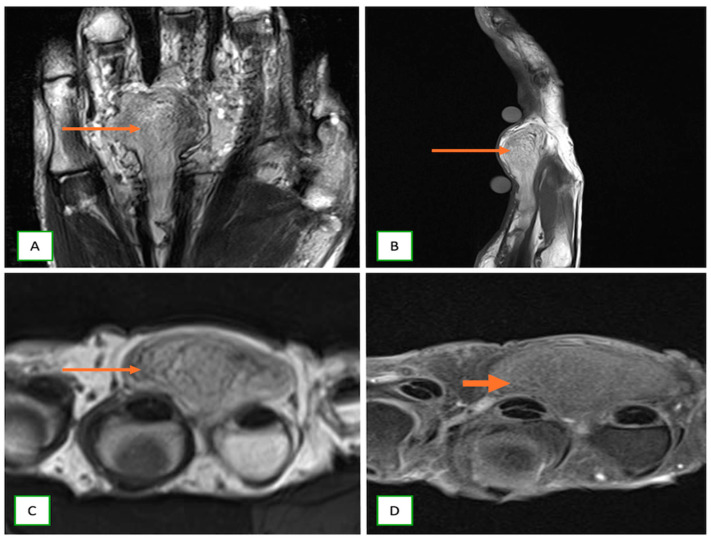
Lipofibromatous hamartoma of a digital nerve in a 53-year-old woman with a lump in their right hand. Coronal T1 (A), sagittal T2 (B), axial T1 (C), and axial fat-saturated T2 (D) fat-saturated T2 images showing the fatty components (thin arrows) appearing hyperintense on both T1 and T2-weighted images with suppression of signal on the fat-saturation images (thick arrows).
